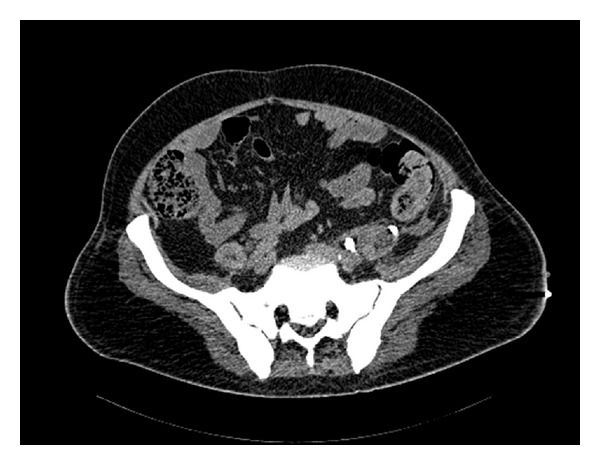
Abdominal CT scan: absence of the urinoma.
